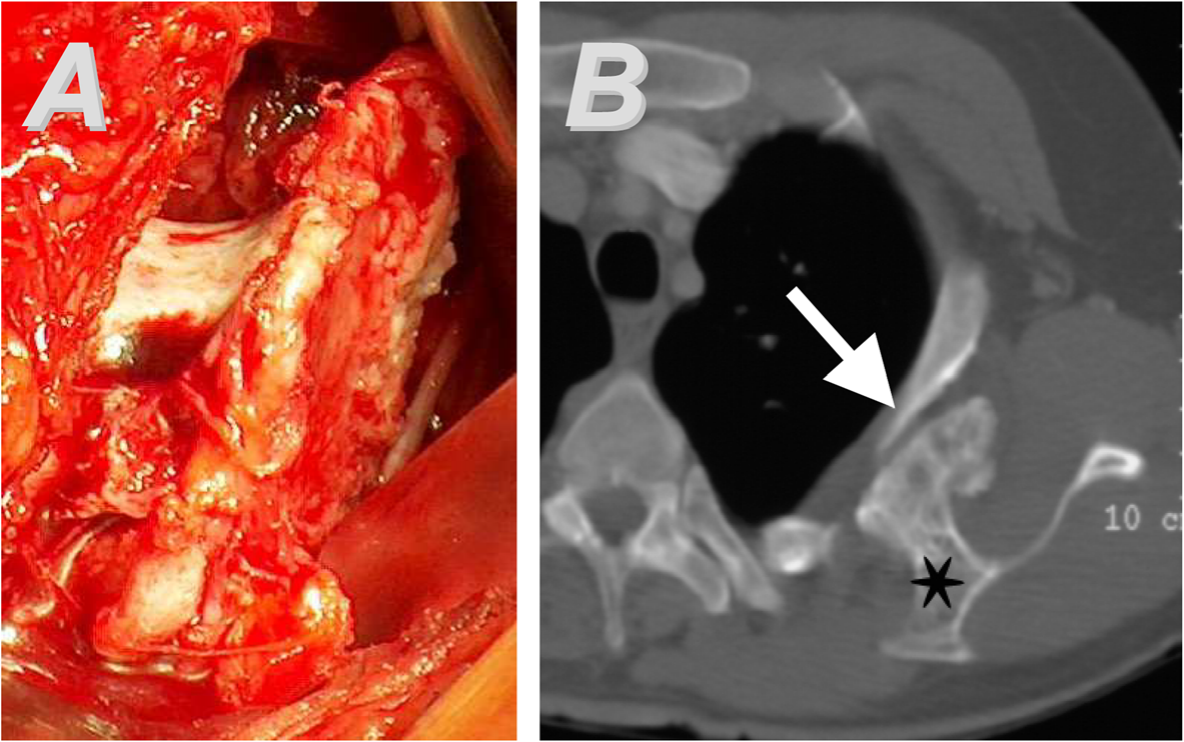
Case 2. The surgical approach to the tumor and the intraoperative finding is shown in (A). The computed tomography (CT) scan (B) demonstrates a bony protuberance (star) protruding from the right scapula, accompanied by a large bursa. The CT image depicts the deformity of the 3rd rib (arrowhead).
